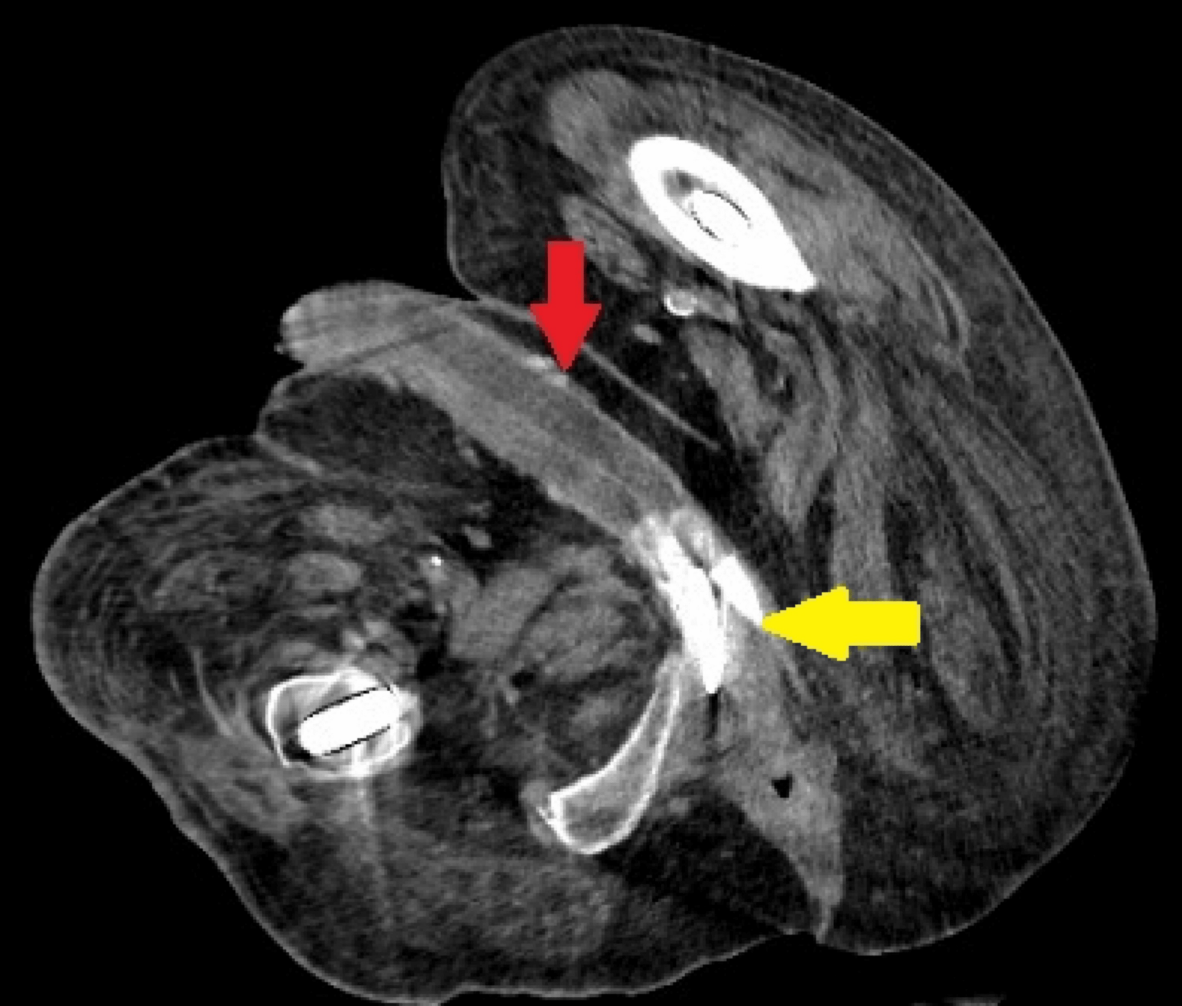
Contrast-enhanced CT scan (axial) of the pelvis The red arrow shows two intracavernosal cylinders surrounded by a small amount of low-attenuation fluid; the yellow arrow shows the two rear tip extenders of the penile prosthesis cylinder.
